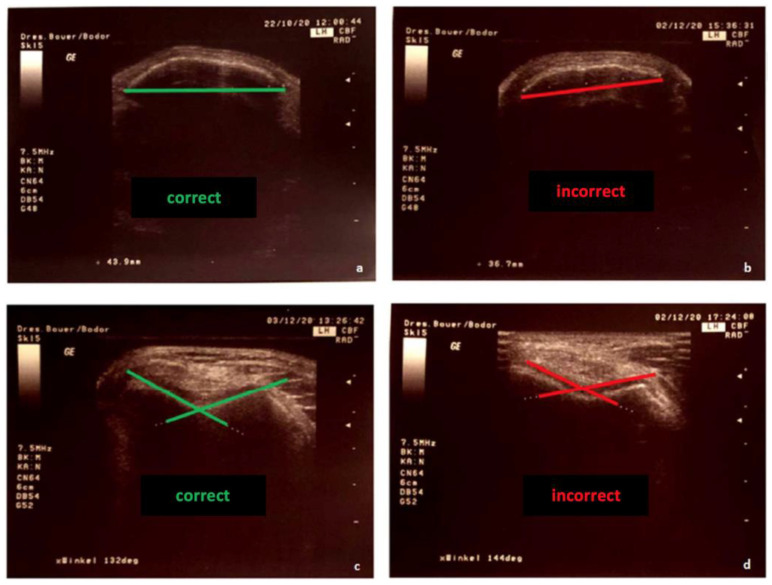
Image settings for sonographic measurement: (a) correct measurement of the patella diameter, (b) incorrect measurement plane, (c) correct positioning of the lines for the angle measurement, (d) incorrect positioning of the lines.
